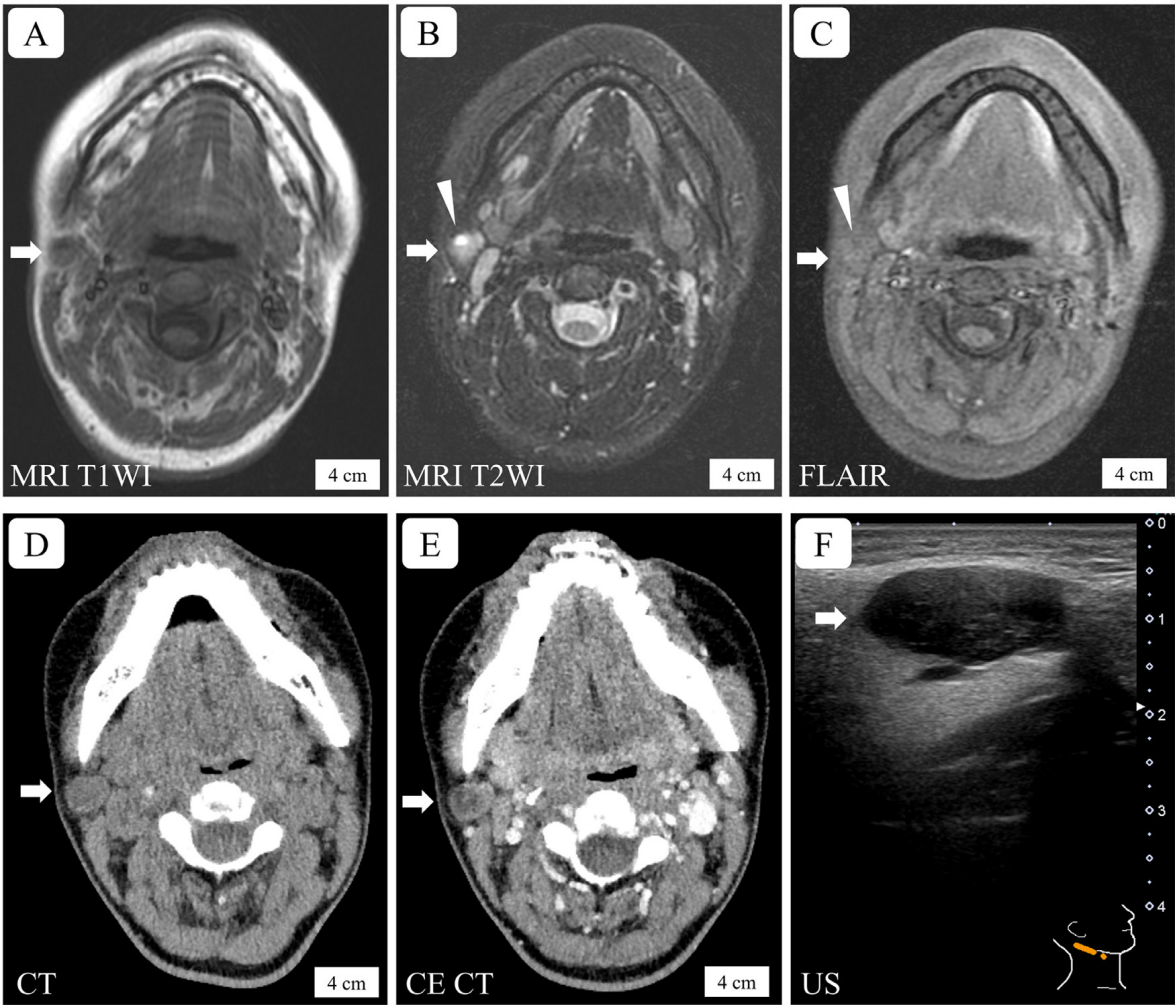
Radiological images of a parotid gland nodule in a young woman with Kimura disease. (A–B) Magnetic resonance images show that the mass has isointensity on a T1-weighted image (A, arrow) and hyperintensity on a T2-weighted image (B, arrow). (C) A fluid-attenuated inversion-recovery image shows an isointense nodule (arrow) with a small low-intensity lesion (arrowhead), which corresponds to a round hyperintense lesion (B, arrowhead) in the nodule on the T2-weighted image. (D–E) Computed tomography images show the nodule is isodense on precontrast imaging (D, arrow) and demonstrates mild heterogeneous enhancement on post-contrast imaging (E, arrow). (F) Ultrasonography performed just before the excisional biopsy demonstrates that the nodule (arrow) is oval and homogeneously hypoechoic. The lengths of the scale bars are shown in the photos. MRI T1 (T2) WI: magnetic resonance imaging T1 (T2) weighted image, FLAIR: fluid-attenuated inversion-recovery, CT: computed tomography, CE CT: contrast-enhanced CT, US: ultrasonography.
